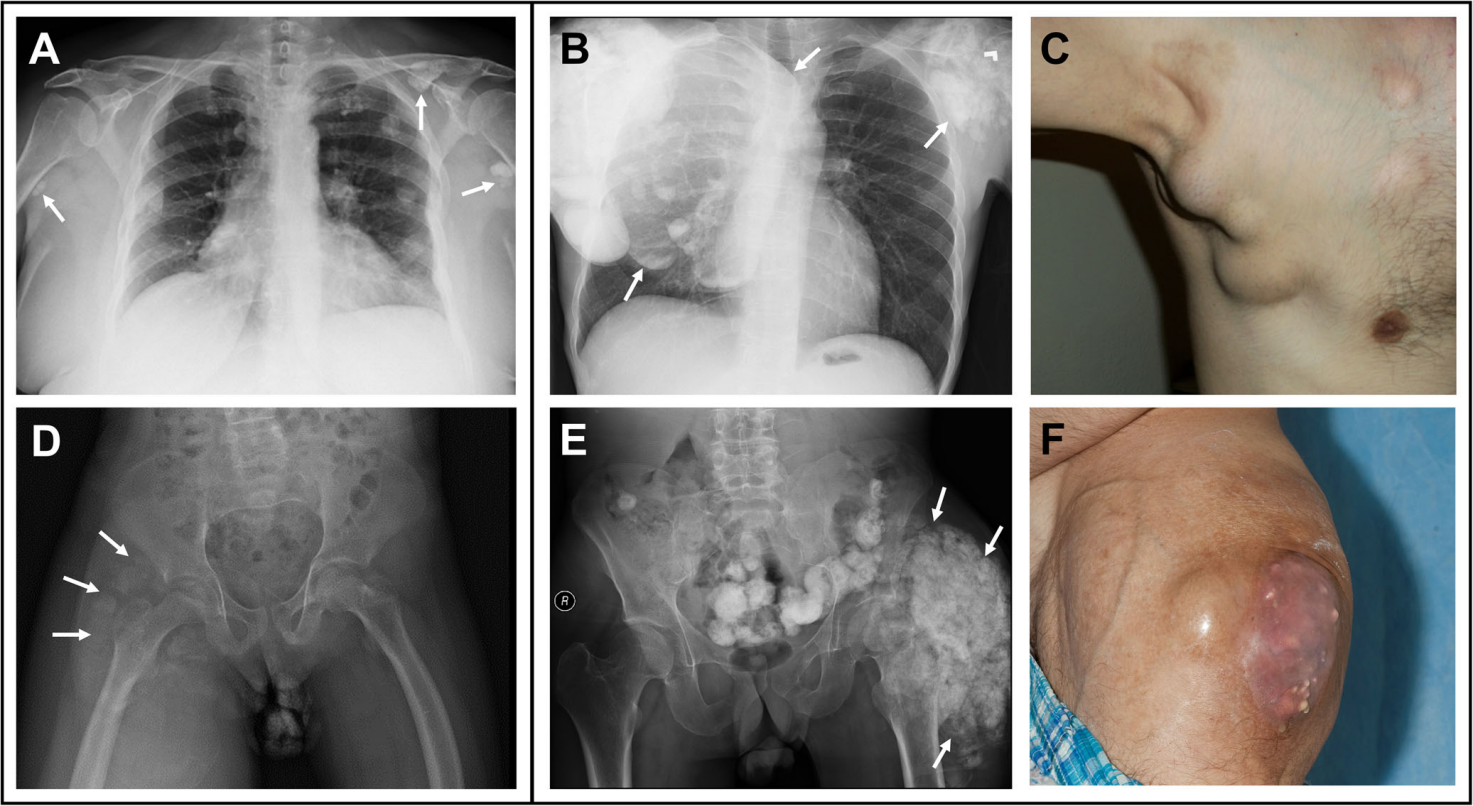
Examples of soft tissue calcifications in hyperphosphatemic familial tumoral calcinosis (HFTC). (*A*) Chest radiograph example of soft tissue calcification (arrows) score 1+ in Table 1. (*B*) Chest radiograph example of soft tissue calcification (arrows) score 3+. (*C*) Gross image of the axilla and chest calcinosis score 3+. (*D*) Pelvic radiograph example of soft tissue calcification (arrows) score 1+. (*E*) Pelvic radiograph shows extensive calcification of the left hip (arrows) receiving score 3+. (*F*) Gross image of hip calcinosis score 3+.
